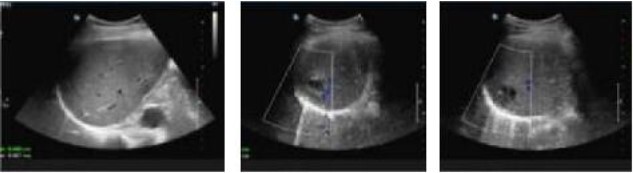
Abdominal color ultrasound findings on the third day after surgery.
